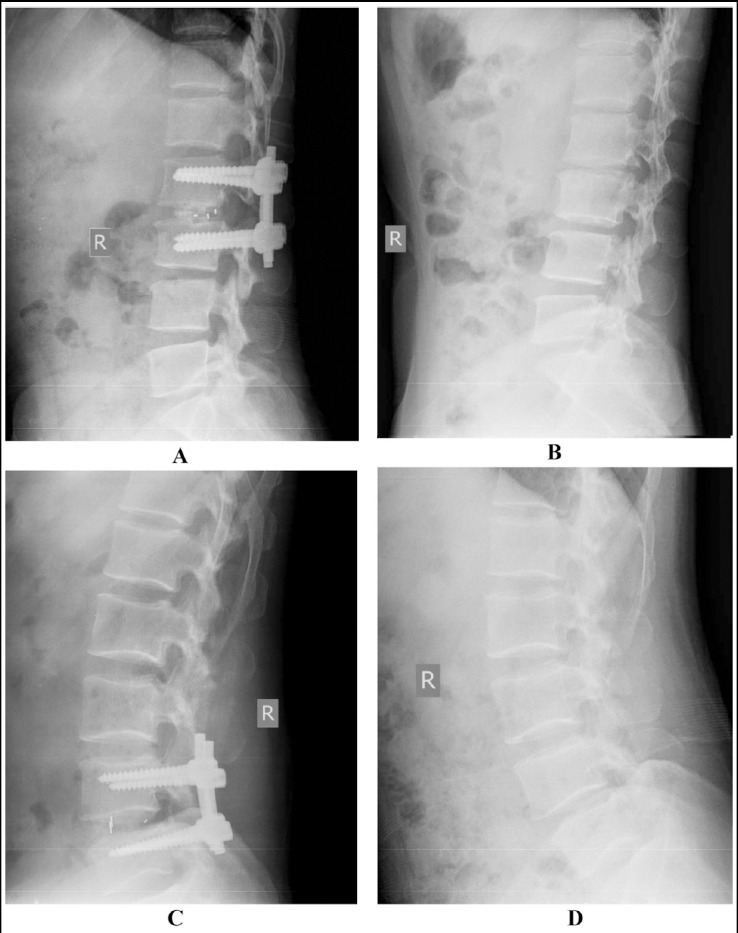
Imaging changes of typical cases before and two years after surgery. A and C: Pre-operative lateral X-ray film; B and D: Lateral X-ray film after bone graft fusion 2 years after operation.
